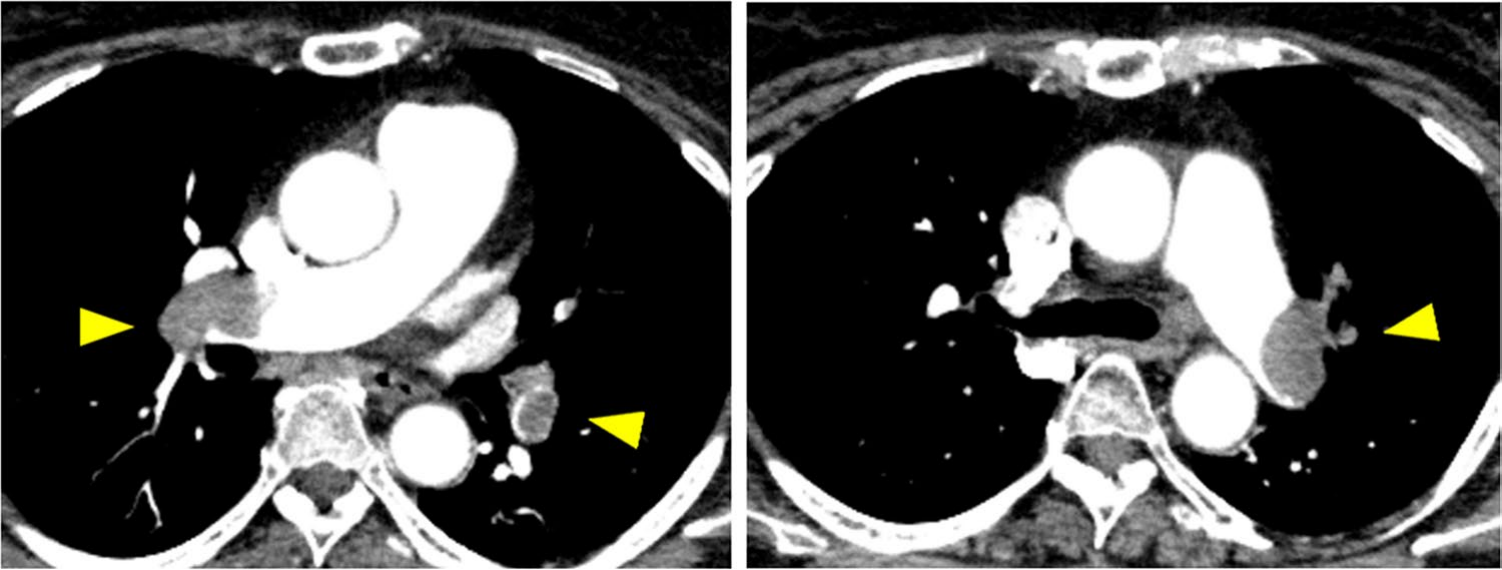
Contrast-enhanced computed tomography reveals prominent emboli in the bilateral main pulmonary arteries (yellow arrowheads)
